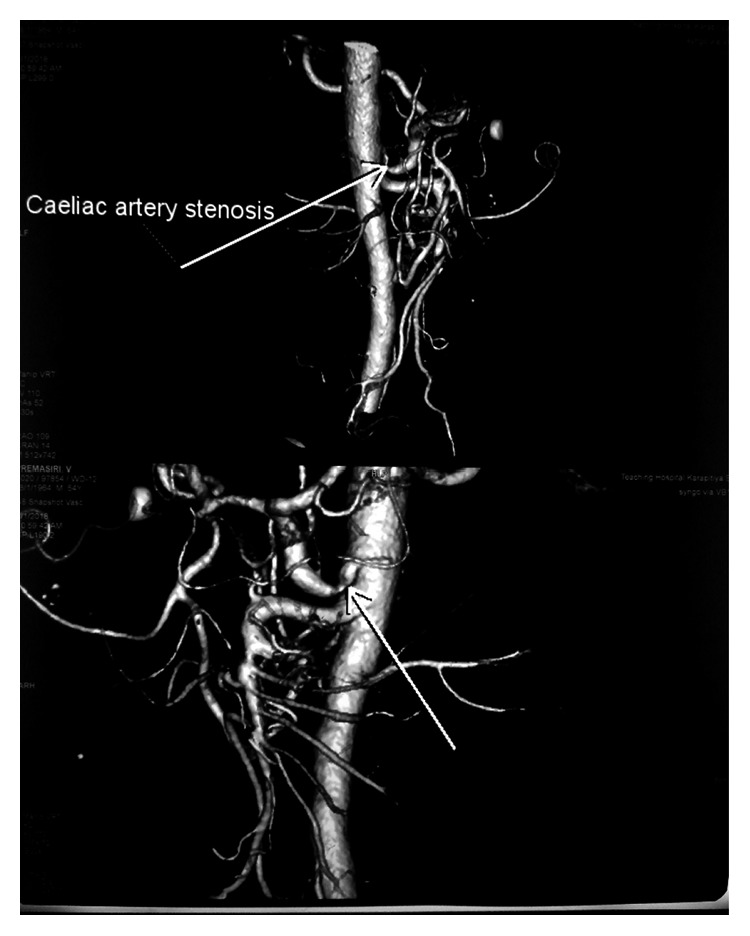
Stenosed segment of the celiac artery in CT angiogram (arrows).
